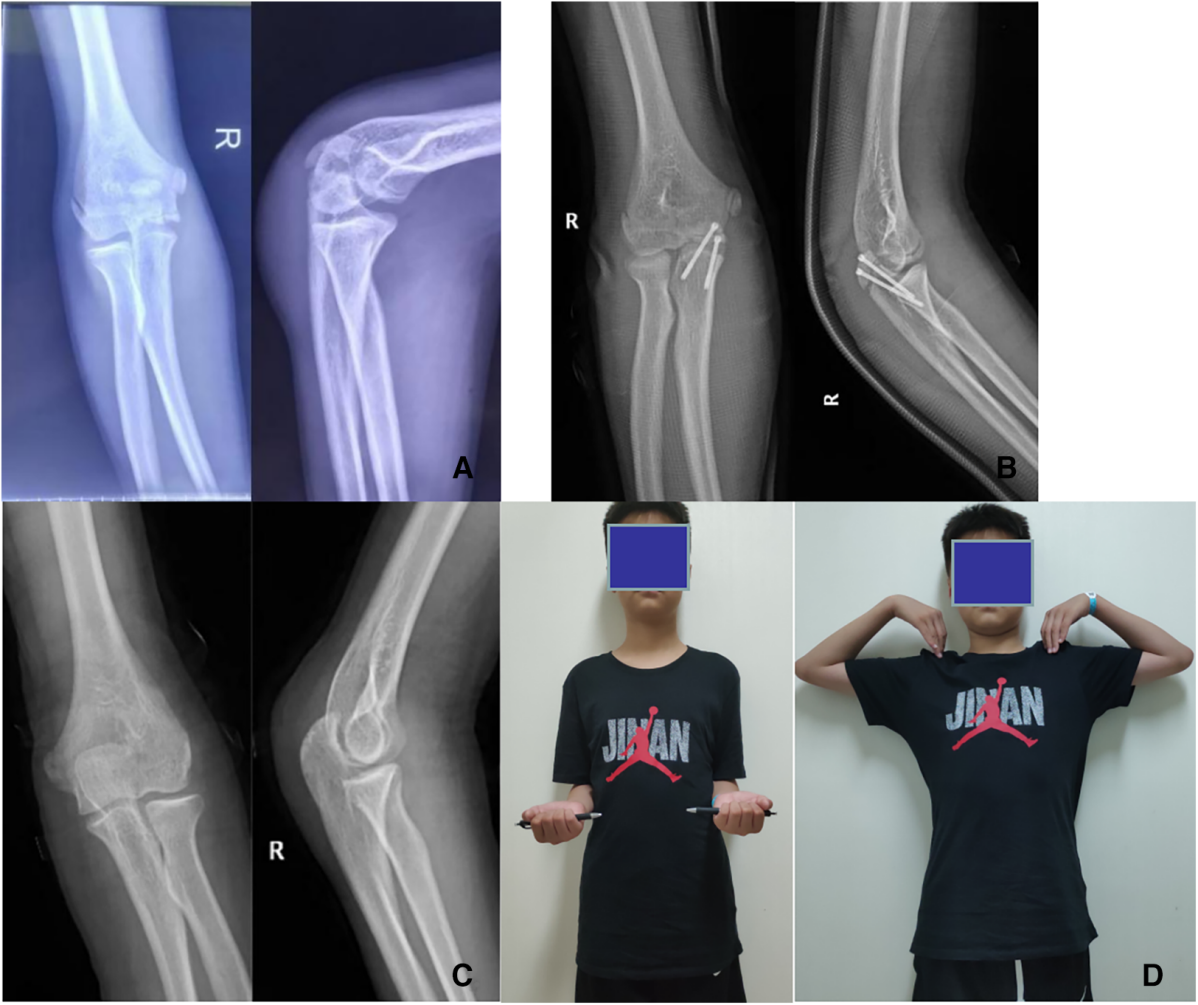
(A) preoperative images; AP and lateral radiographs in a 13-year-old boy with an olecranon fracture (B) postoperative images from the 1-day follow-up and AP and lateral radiographs in the same patient treated with closed reduction and Herbert screw fixation. (C) Follow-up images obtained at 6 months after surgery in the same patient. (D) Functional outcomes in the same patient at 6 months after surgery. Before removing the cannulated screw, we recorded the patient's elbow range of motion in flexion, extension, pronation, and supination, forearm length, and QuickDASH score.
